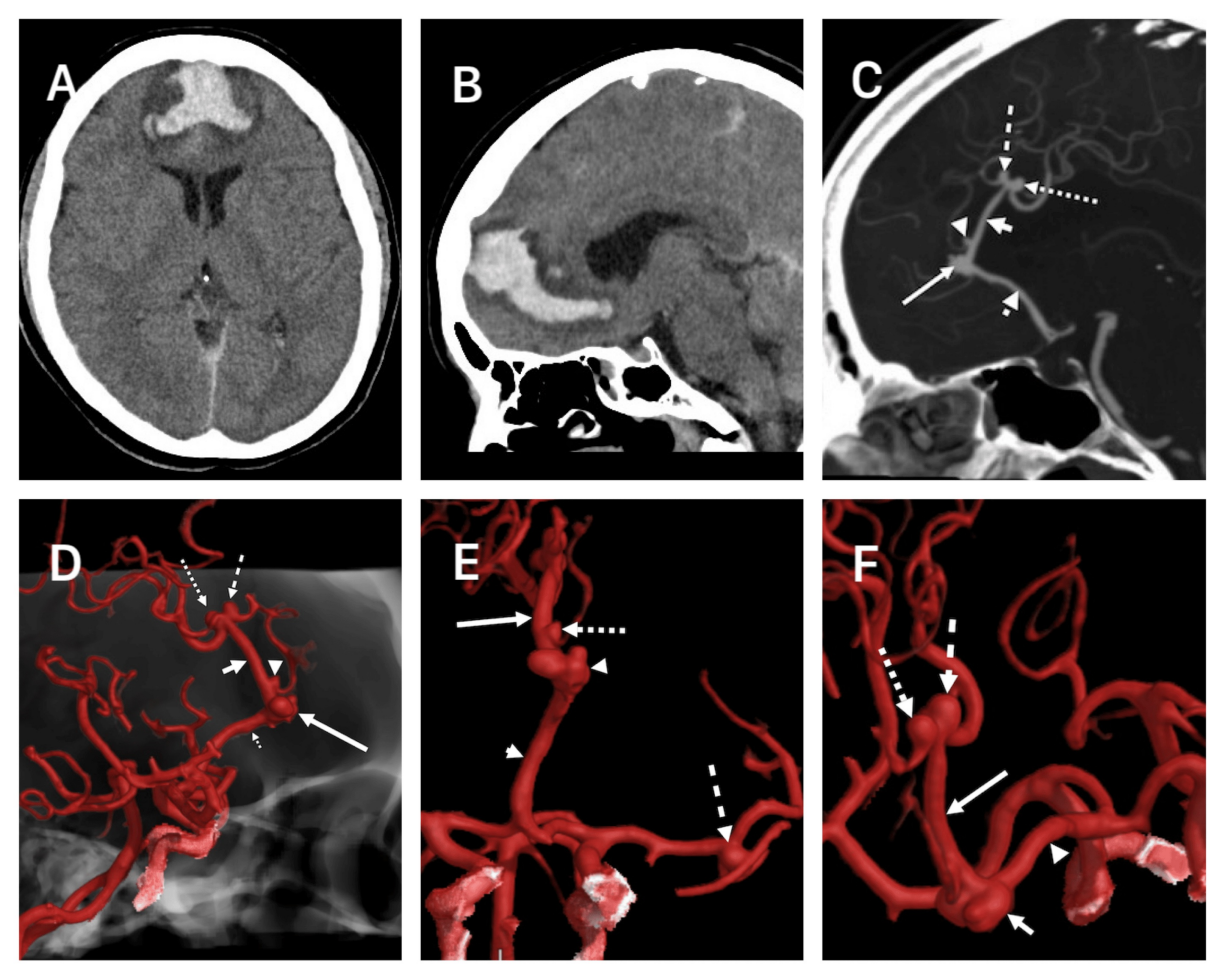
CT images and 3D reconstructed angioCT. (A) Axial CT showing an interhemispheric-frontal hematoma. (B) Sagittal CT showing the interhemispheric hematoma extending subcallosally. (C) Sagittal AngioCT evidencing four DACAa, an anterior A3 (large arrow), two “kissing” superior A3 (dashed and dotted arrows), and a small A3 between the anterior and superior A3 (arrowhead); azygos A2 ACA variation (dotted arrowhead) and the single A3 pericallosal artery ACA variation (small arrow). (D) Lateral angioCT reconstruction showing multiple DACAa, the large anterior A3 (long arrow), “kissing” superior A3 (long dashed and dotted arrows), small A3 (arrowhead), azygos (short dotted arrow), and the single pericallosal A3 artery (short arrow). (E) Anteroposterior angioCT showing the azygos (short arrow), single pericallosal artery A3 trunk (long arrow), and three aneurysms, the large anterior A3 DACA (arrowhead), the small A3 DACA (dotted arrow), and the MCA (dashed arrow). (F) Superior view angioCT of the “kissing” superior A3 DACAa (dashed and dotted arrows), single A3 pericallosal artery (long arrow), large lobulated anterior A3 DACAa (short arrow), and the azygos variant. CT: computed tomography, DACAa: distal anterior cerebral artery aneurysm, ACA: anterior cerebral artery, MCA: middle cerebral artery.
